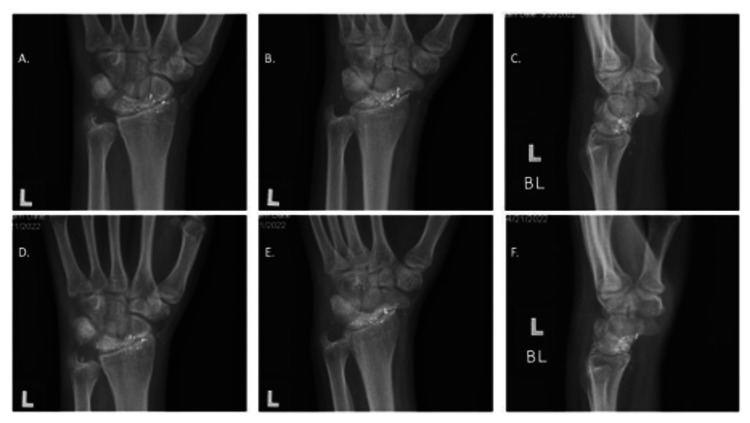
Postoperative plain radiographs. 10 days post-op anteroposterior and lateral X-Ray images (A-C). 5 week post-op anteroposterior and lateral X-Ray images (D-F).
